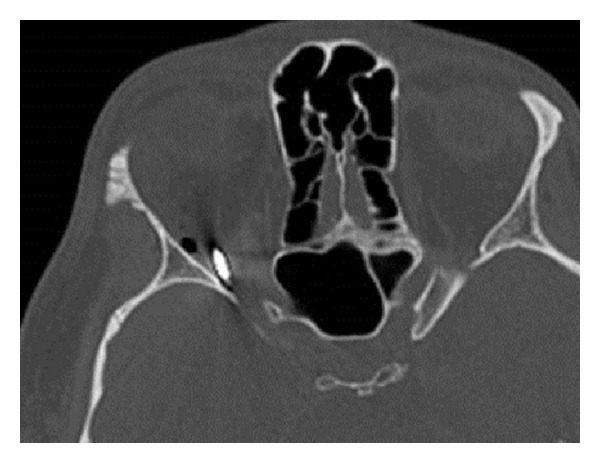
Horizontal head CT showing a metallic foreign body in the right apex.
